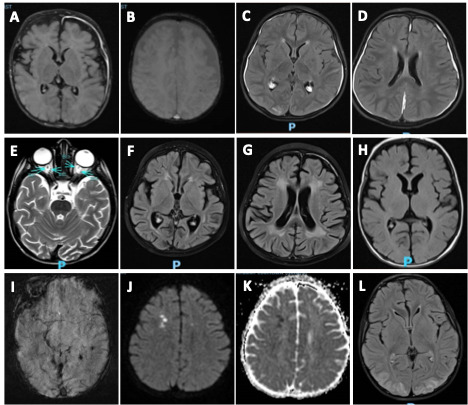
MRI abnormalities in pediatric patients with primary HLH. A,B) 1-month old boy with heterozygous STXBP2 mutation with initial normal MRI and subsequent post contrast axial T2 FLAIR imaging showing mild cerebral atrophy with left cerebral subdural effusion. Susceptibility weighted image shows punctate petechial hemorrhage. C-E) 23-month-old boy with homozygous pathogenic STX11 mutation with initial post-contrast axial T2 FLAIR images showing subdural fluid collection, right occipital dural enhancement and optic nerve sheath enhancement. F, G) 2 months later, post contrast axial T2 FLAIR images show cerebral volume loss and white matter T2 prolongation. H, I) 23-day old baby boy with compound heterozygous frameshift PRF1 mutation with axial T2 and SWI images showing left peri-rolandic gray matter involvement with focus of left temporal punctate hemorrhage. J-L) 3-month-old baby girl with pathogenic UNC13D mutation with DWI and ADC images showing diffusion restriction which
